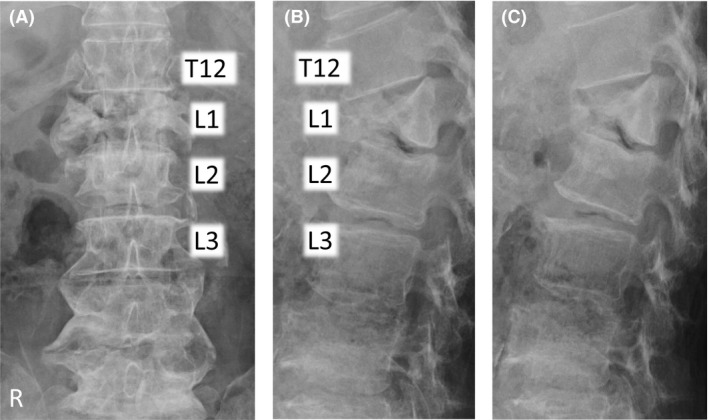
Plain radiographs in decubitus position. (A) Anteroposterior view, (B) lateral view in the flexion position, and (C) lateral view in the extension position on plain radiography performed on the examination table. These showed an L1 vertebral body fracture with an intact posterior wall. Flexion and extension views in the decubitus position showed no obvious malalignment of the vertebrae. Endplates of both sides were fractured without involvement of the posterior wall of the vertebral body; this was classified as AOS type A2.
